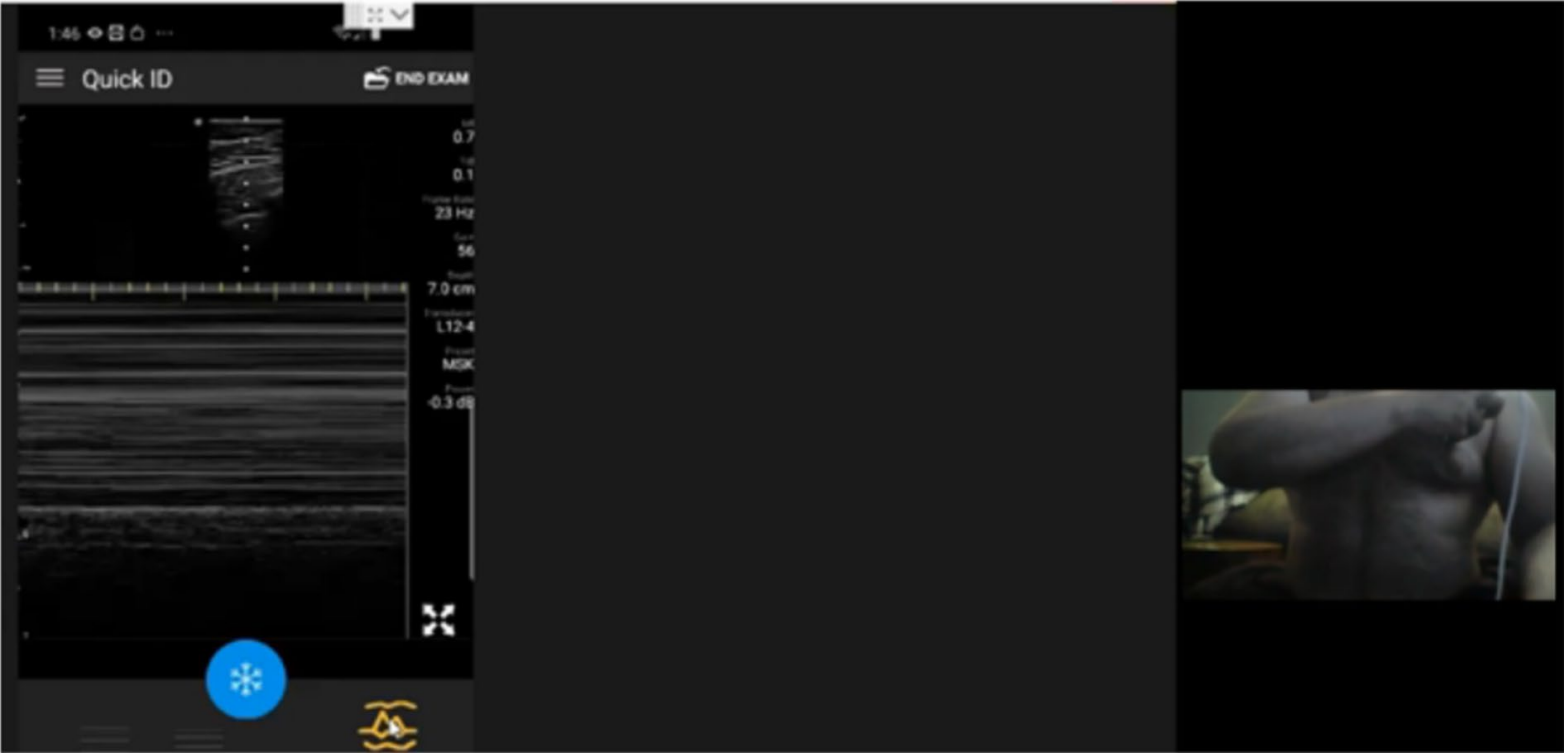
Second follow-up Self-Performed Lung Ultrasound Study of 32-year-old patient 2 weeks 2 days after COVID-19 diagnosis. Screenshot of the Remote Mentors Computer Screen that shows the patient imaging himself to the right of the screen and the resultant ultrasound image showing the “seashore” sign to the left. As lung ultrasound is a dynamic real-time examination hard to capture in any single image, readers are strongly encouraged to view the accompanying supplemental videos of the entire examination online to gain a true appreciation of the technique
